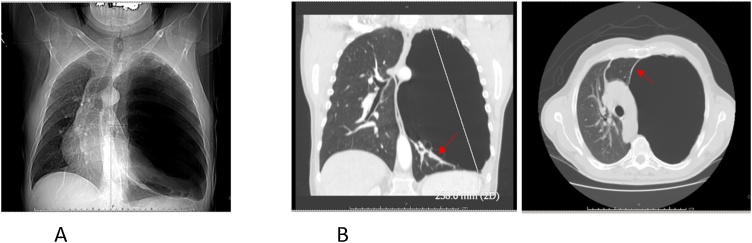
A. Preoperative chest X-ray; 1B Chest-CT showing a giant bulla on the left side, displacing the mediastinum on the right. Arrows indicate the double-wall signs.
